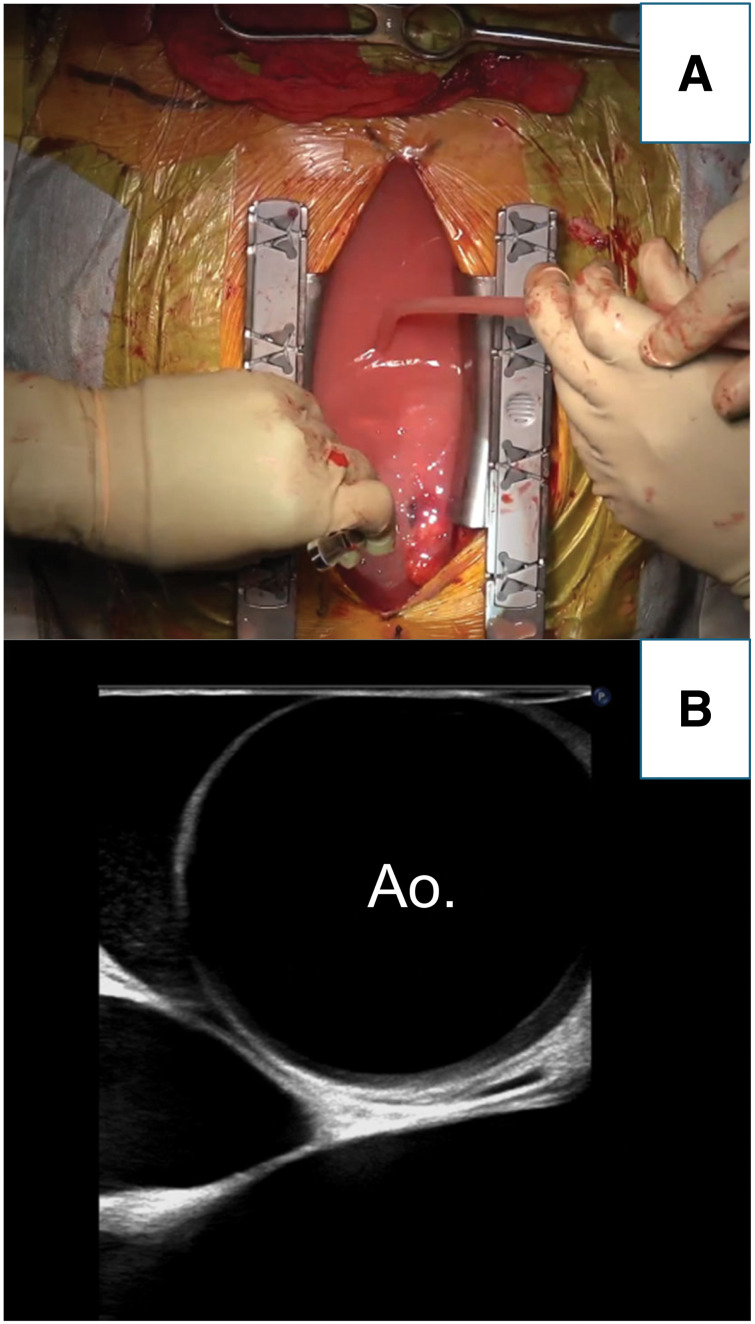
(A) Chylous pericardial effusion drained via pericardiotomy. (B) The echocardiogram performed during surgery did not reveal any signs of aortic dissection (ascending aorta: Ao.).
